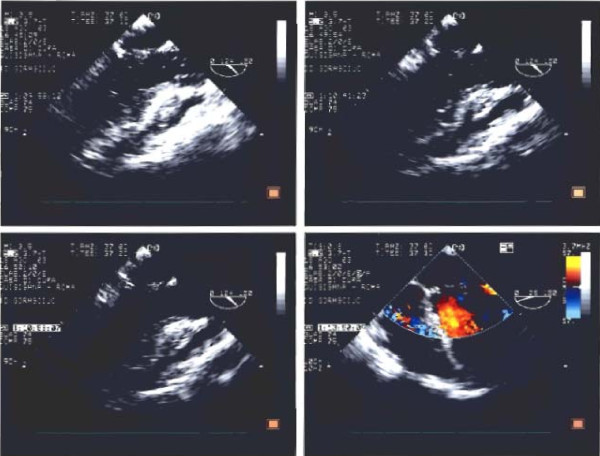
Transesophageal echocardiographic view of mitral valve: valve dysplasia and anterior leaflet cleft are showed.
